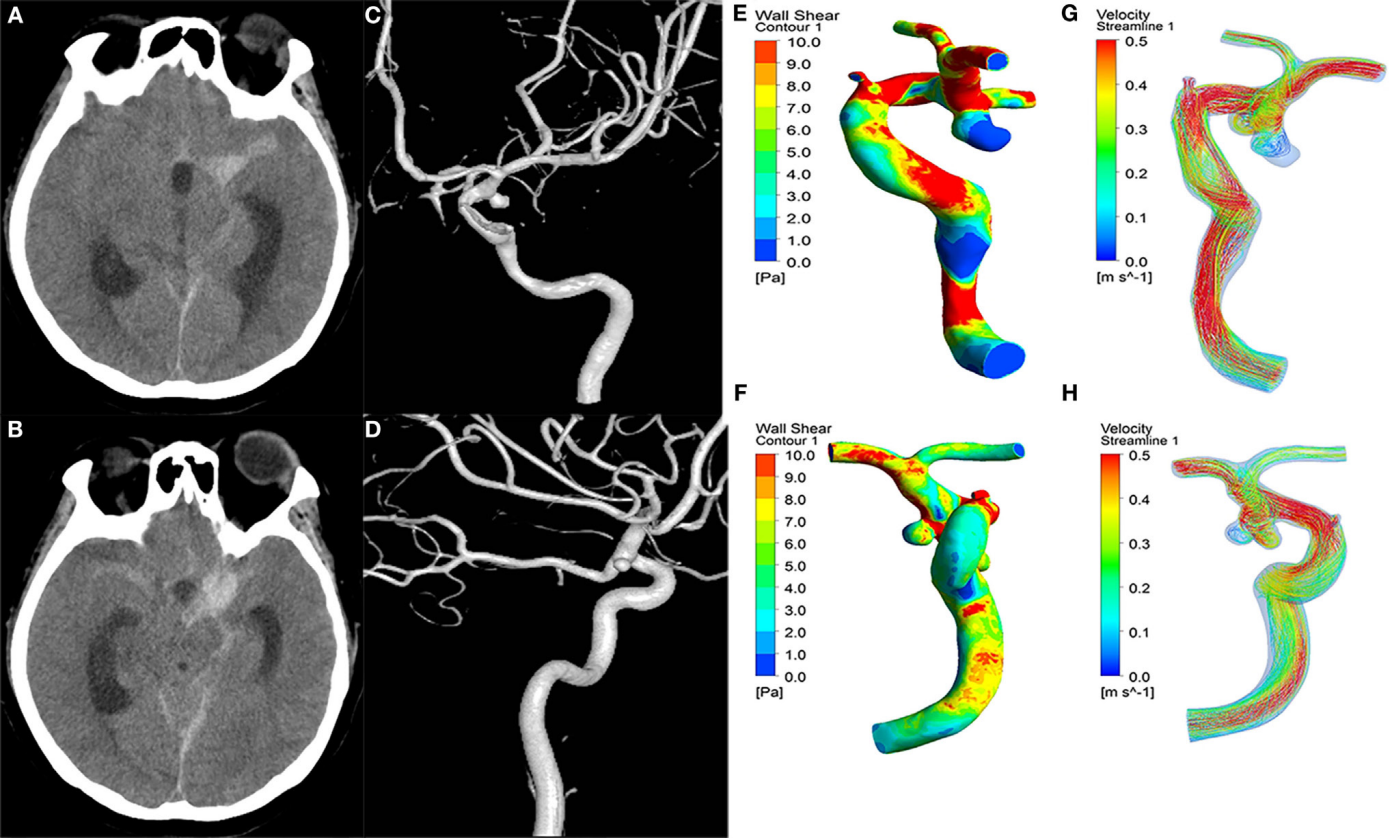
A 43-year-old female manifested severe headache. First column (A,B): CT showed subarachnoid hemorrhage. Second column (C,D): the 3D-DSA images presented with two aneurysms on the left ICA C7 (ruptured) and right ICA C7 (unruptured). Third column (E,F): wall shear stress (WSS) distribution. The ruptured aneurysms (E) had significantly lower WSS than the unruptured aneurysms (F). Fourth column (G,H): velocity streamlines showing the flow pattern of the aneurysms. The flow patterns of the two aneurysms were simple at peak systole.
